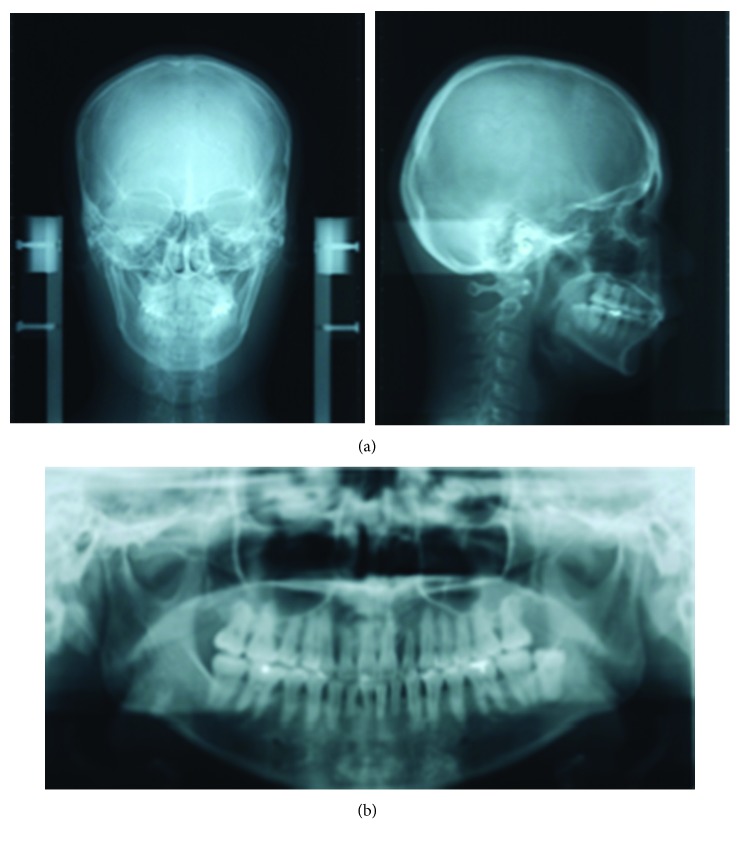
A post-retention cephalograms (a) and panoramic radiographs (b) are shown.
